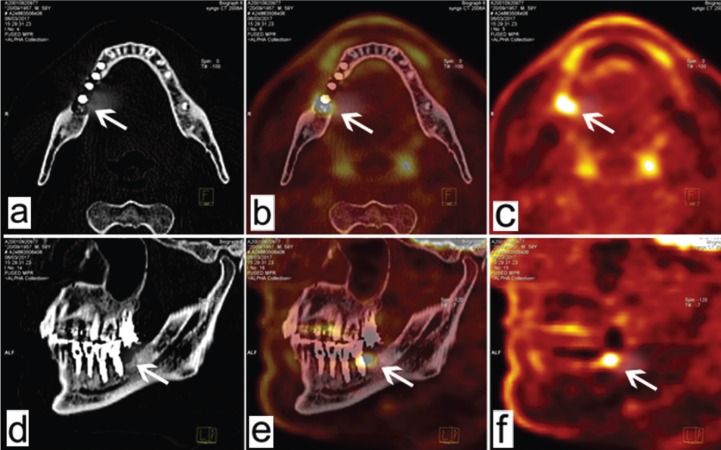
Patient 2. Reconstructed FDG PET/CT images acquired parallel to the occlusal plane (a, b, c) and parasagittally (d, e, f). Position 47 (arrows) shows clinical peri-implant hypermetabolism, whereas the other implant sites are normal.
